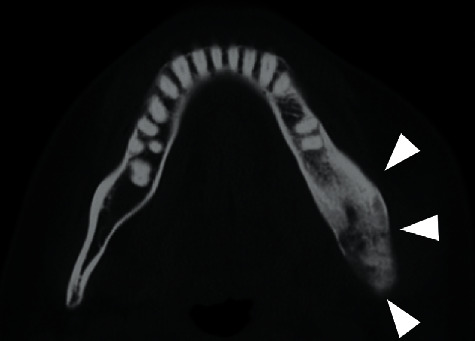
Computed tomography showing a bulge with an osteosclerosis was observed from the left mandibular branch to the mandibular condyle (arrowhead).
